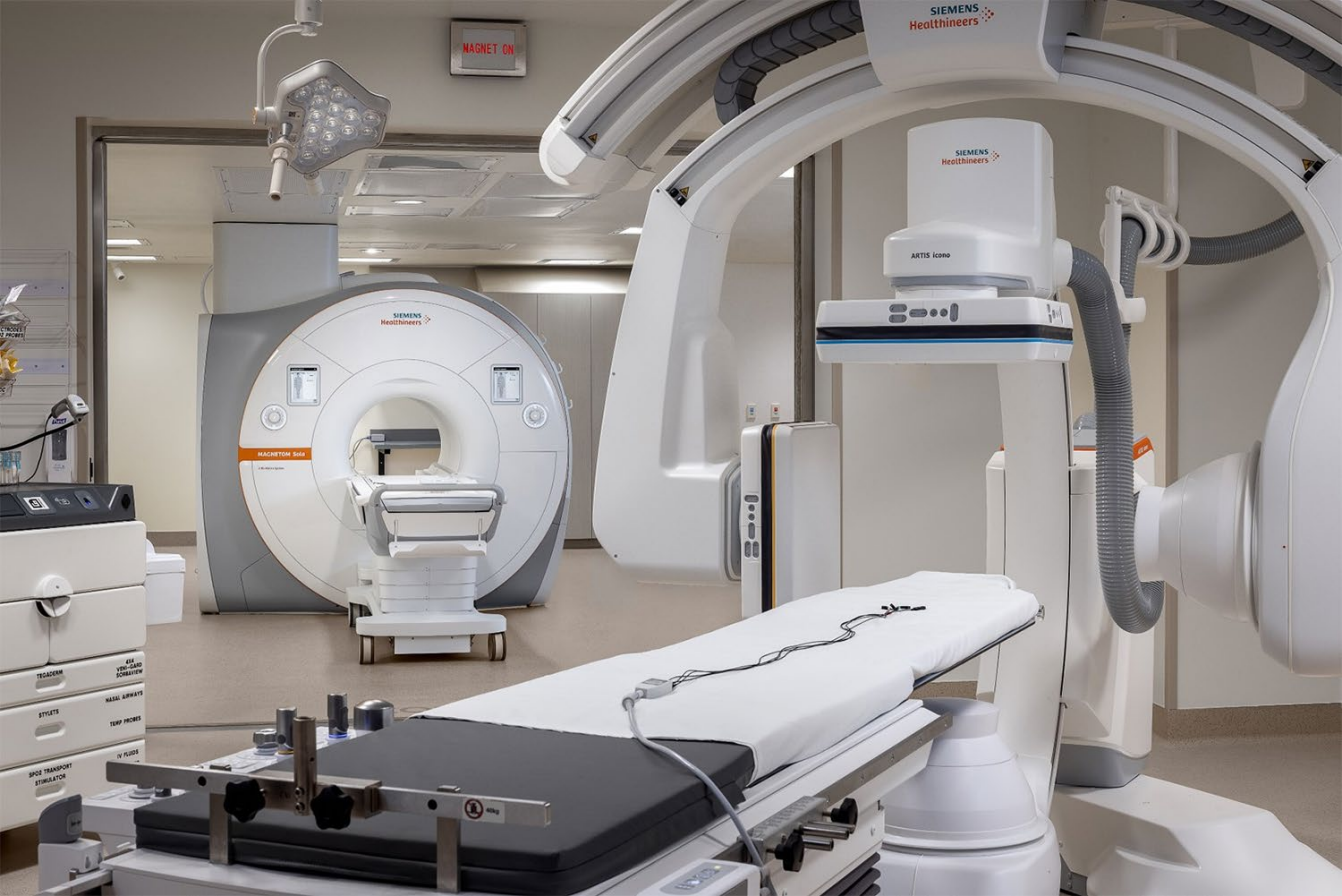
iCMR suite at Rady Children’s Hospital, University of California - San Diego. A co-localized biplane X-Ray fluoroscopy room and 1.5T cardiac MRI room are separated by radiofrequency shielded sliding doors allowing independent and combined room use. A second biplane X-Ray fluoroscopy room is adjoining a room to house a future imaging modality also intended for independent and combined room use
